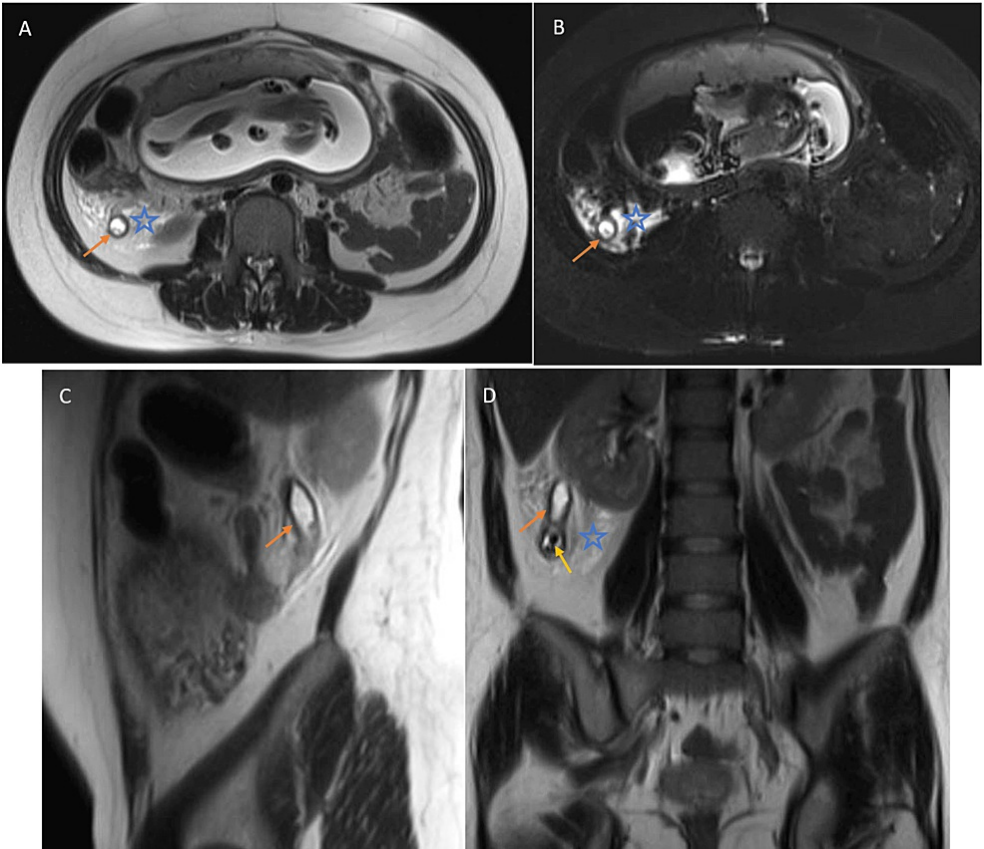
Appendicitis on T2W sequences T2-weighted (T2W) (A, C, D) and T2W half-Fourier single-shot turbo spin-echo (HASTE) short tau inversion recovery (TIRM) (B) images of a proven case of acute appendicitis in a 32-year-old woman at a 20-week gestation with acute epigastric pain migrating to the right iliac fossa. The dilated and fluid-filled inflamed appendix was well visualized (orange arrow). Appendicolith (yellow arrow) and peri-appendiceal stranding (star) were also apparent.
